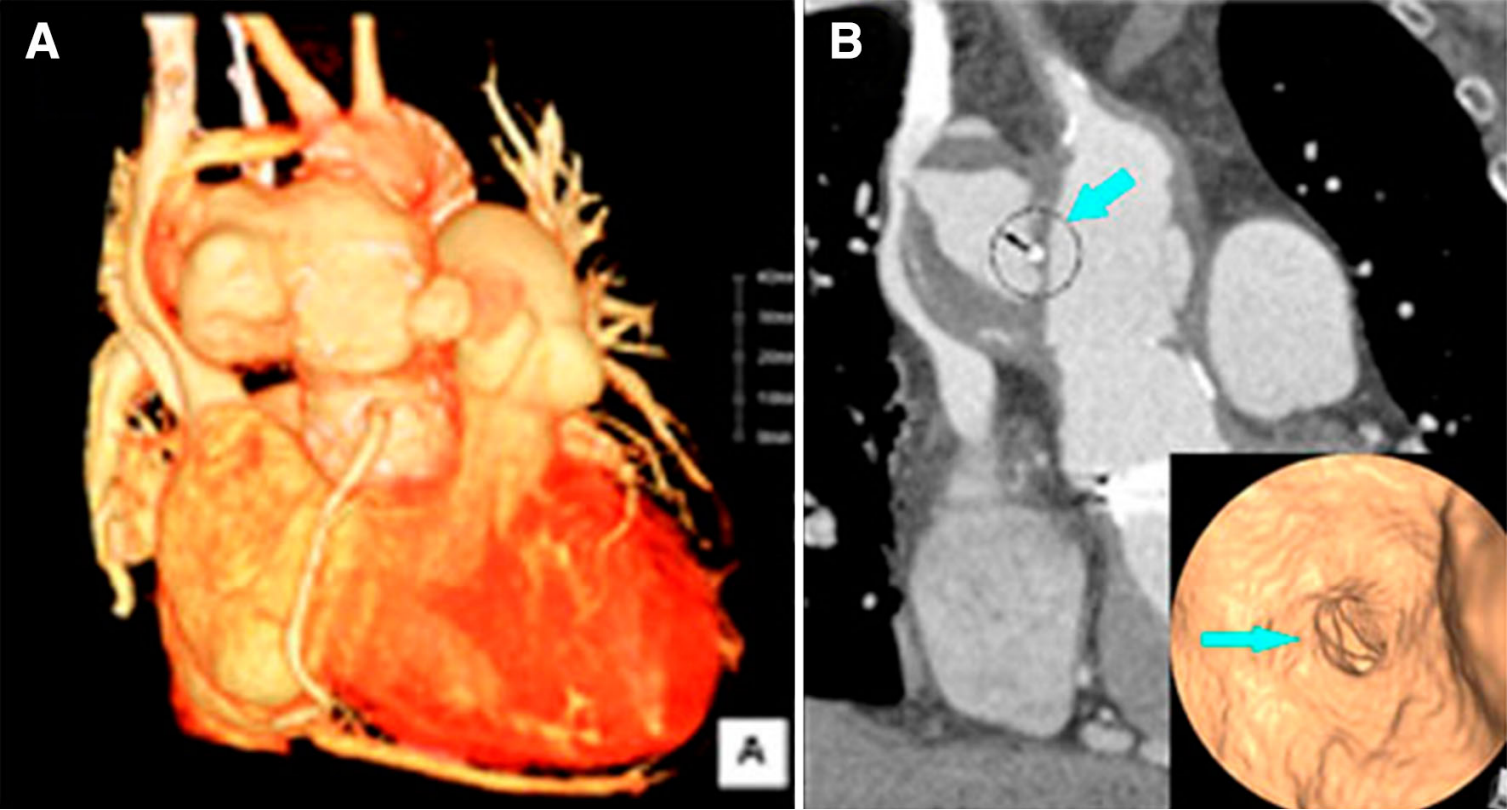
a A 73 × 44 mm large false aneurysm with an irregular shape was found, and the false aneurysm compressed the superior vena cava (SVC). b Coronal CT and a virtual CT endoscopic view showed the prosthetic graft punch hole (*arrows*)
